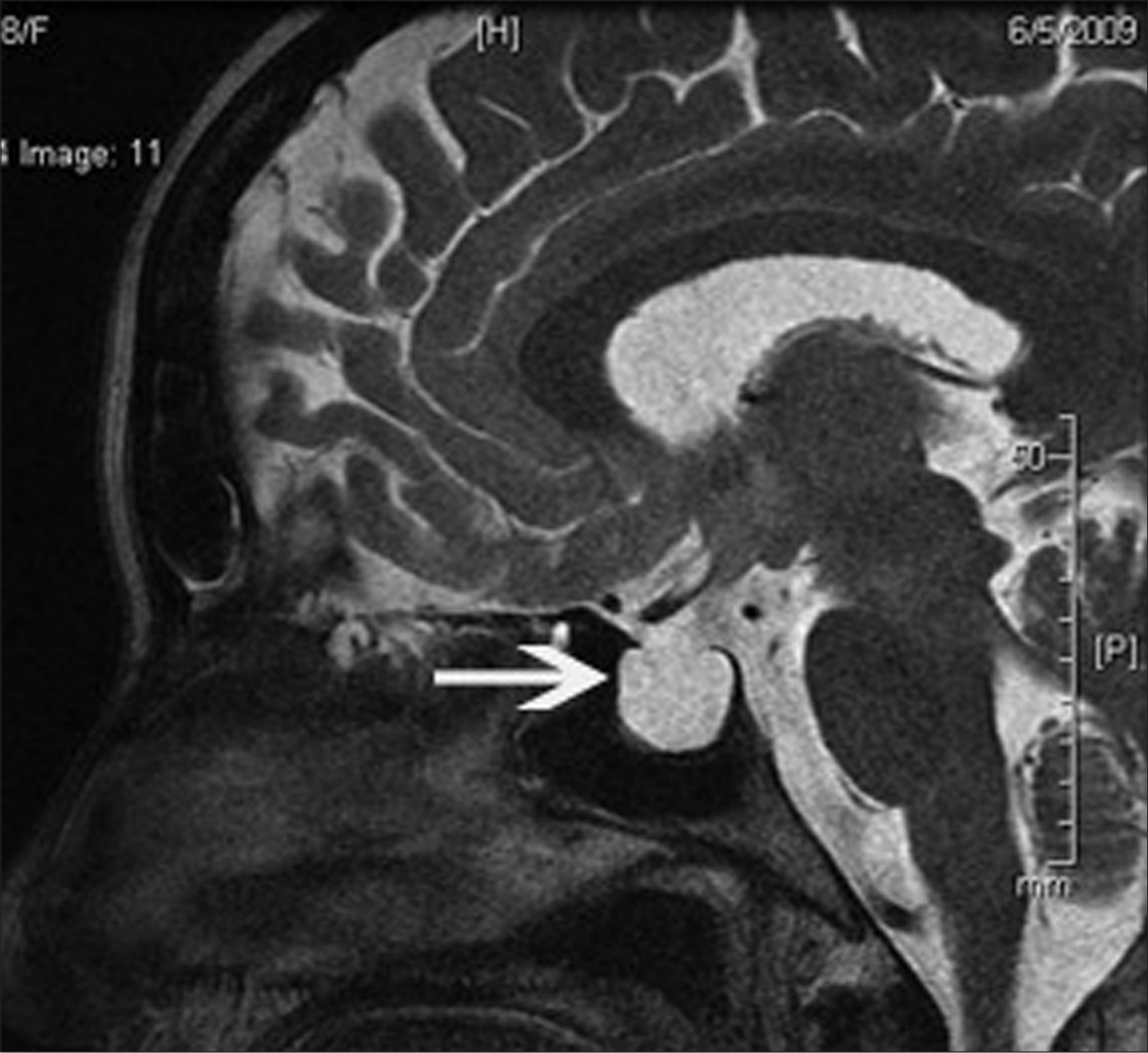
Sagittal T2-weighted image shows complete empty sella (white arrow)
